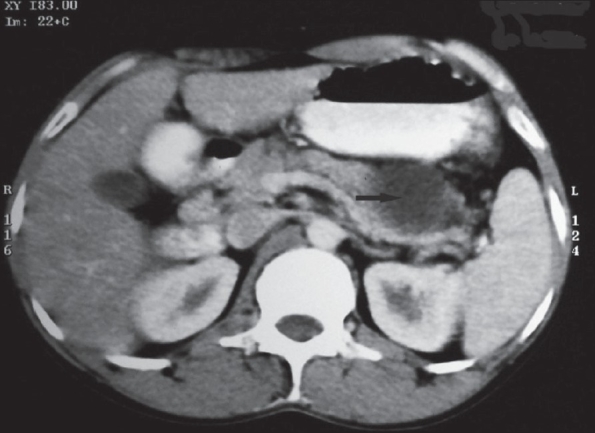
CT scan abdomen after oral and IV contrast showing a cystic lesion in the region of tail of pancreas suggestive of pancreatic pseudocyst
